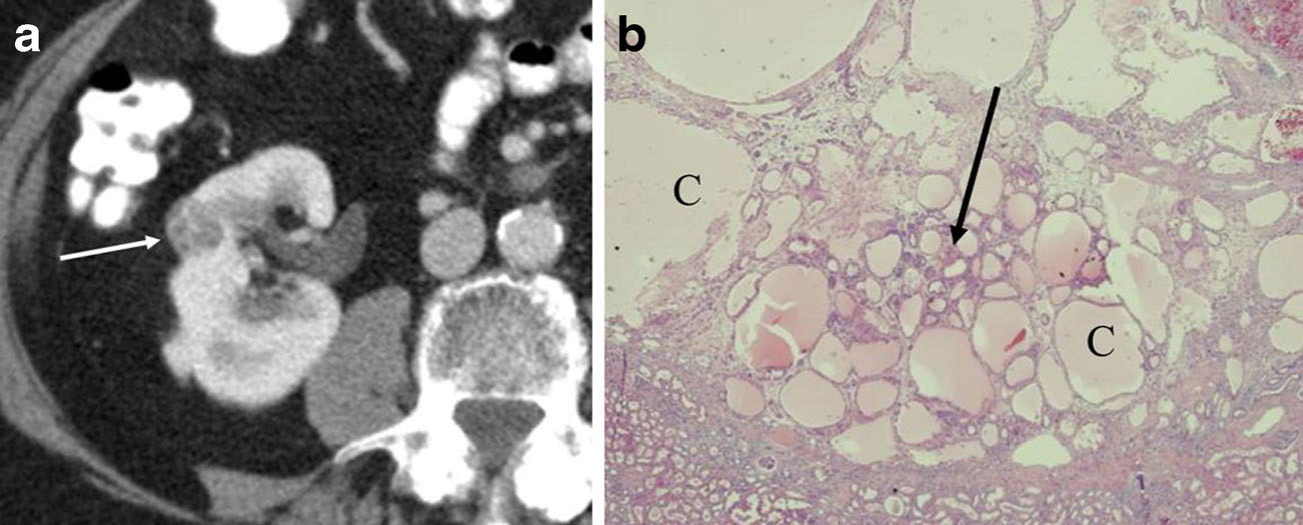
An 80-year-old woman with a right renal oncocytoma with cystic change. a The corticomedullary phase of the axial CE-CT demonstrates a small multilocular cystic right renal mass (*arrow*). b (Low magnification) Oncocytoma, tubulocystic pattern, tumour cells arranged as variably-sized cysts (*C*) and tubules (*arrow*)
